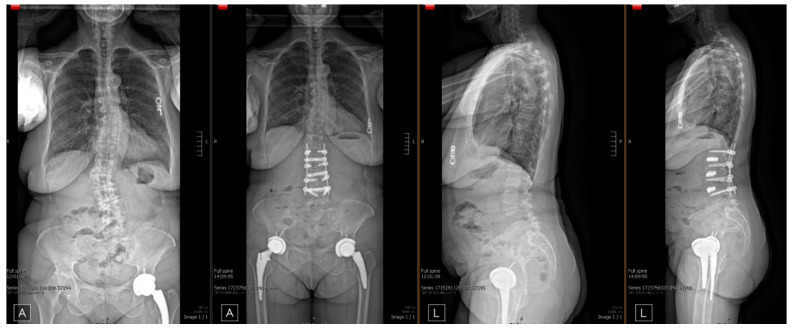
Pre- and postoperative standing radiographs. Example radiographs demonstrating accurate hardware placement and restoration normal spinal alignment following AR-guided L2–5 LLIF with L2–5 PSF.
